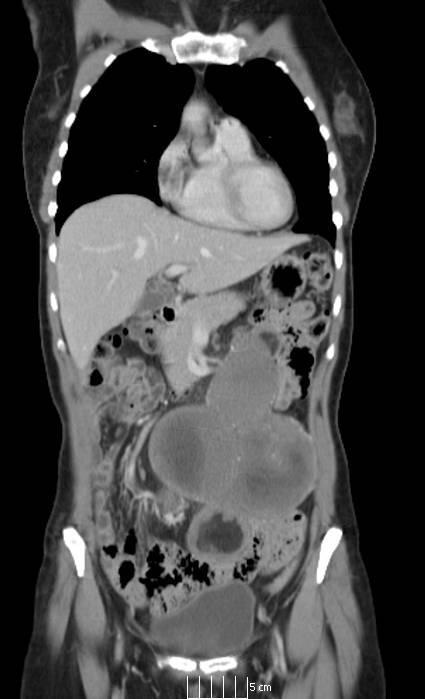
Coronal computed tomography images showing multiple solid and cystic intra-abdominal lesions marked with an arrow with associated calcification in the bowel wall.
